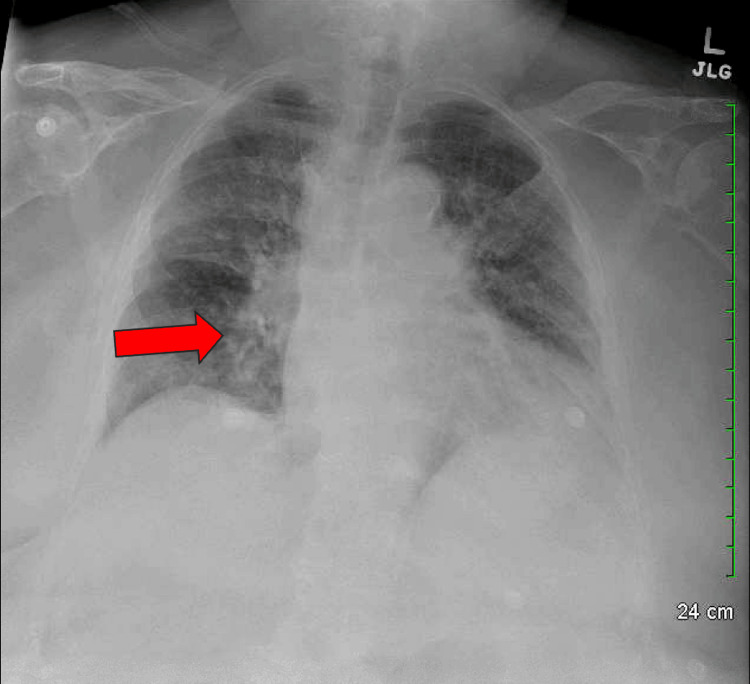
Chest X-ray on admission. The arrow indicates cardiomegaly and bilateral perihilar opacities, consistent with pulmonary edema.
